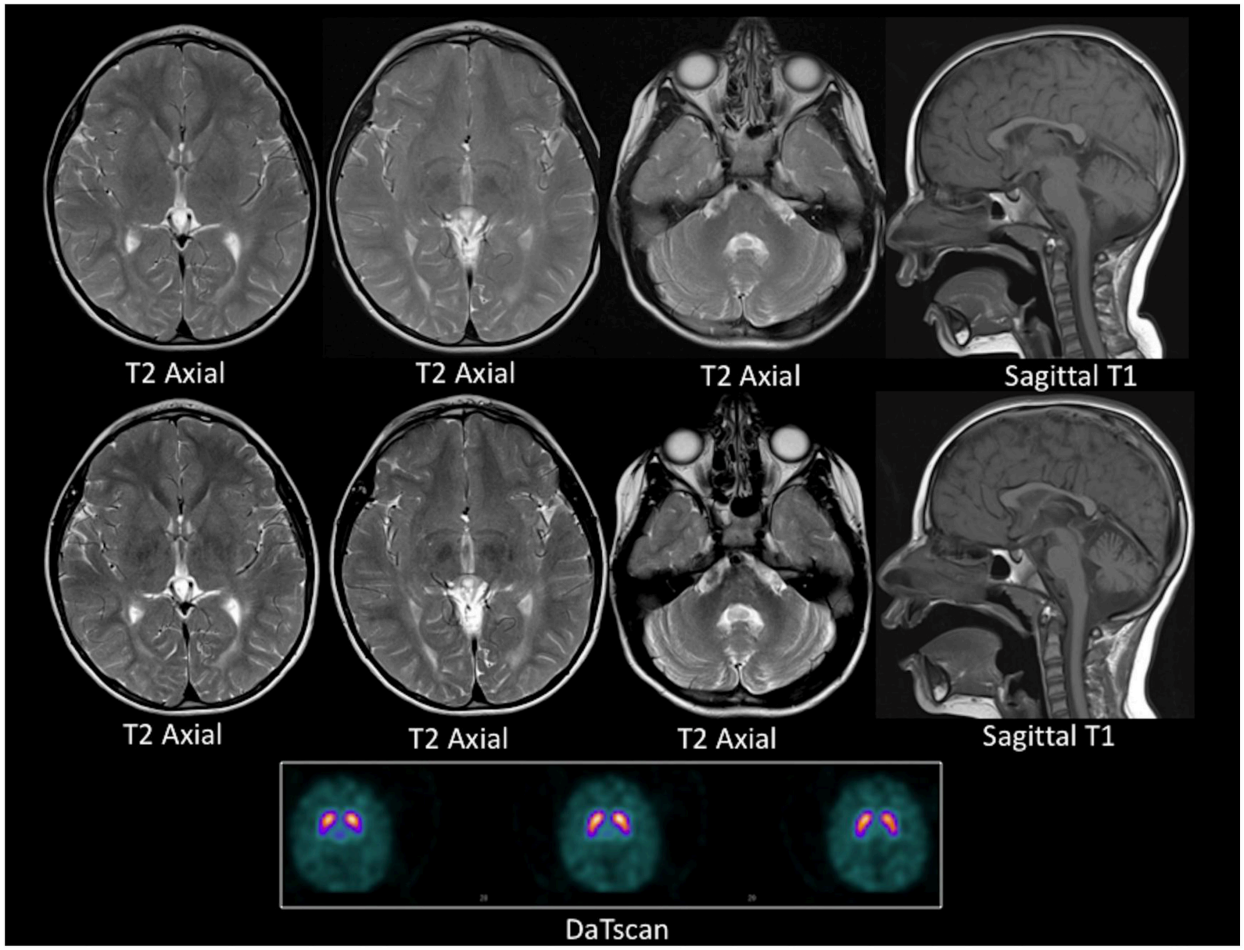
MRI and DaTSCAN images. Top row: Axial T2 weighted images and Sagittal T1 weighted image at age 4 years. Middle row: Axial T2 weighted images Sagittal T1 weighted image at age 5 years. Lower panel: DaTSCAN. All MR images were acquired on a 1.5T scanner. The top and the middle row images show progressive iron deposition in the globus pallidi, more than expected for age, and consistent with neuronal brain iron accumulation. There is global paucity of the cerebral white matter with a thin corpus callosum. There is also cerebellar atrophy between the two time points. The DaTSCAN is normal.
